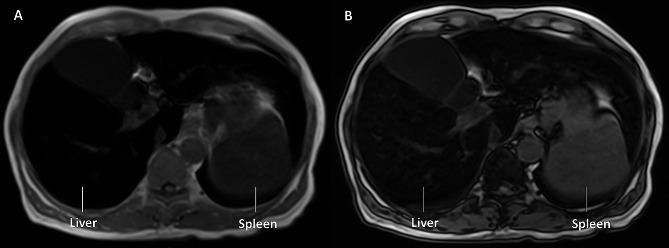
Dual-sequence magnetic resonance imaging (MRI) of the proband with *SLC40A1*-related haemochromatosis. The T1-weighted imaging displayed hypo-signal intensity in the liver and spleen, and the signal intensity of liver was lower than that of spleen. The signal intensity of the liver at axial gradient-echo (GRE) T1-weighted in-phase sequence (A) was lower than that at the out-of-phase sequence (B)
